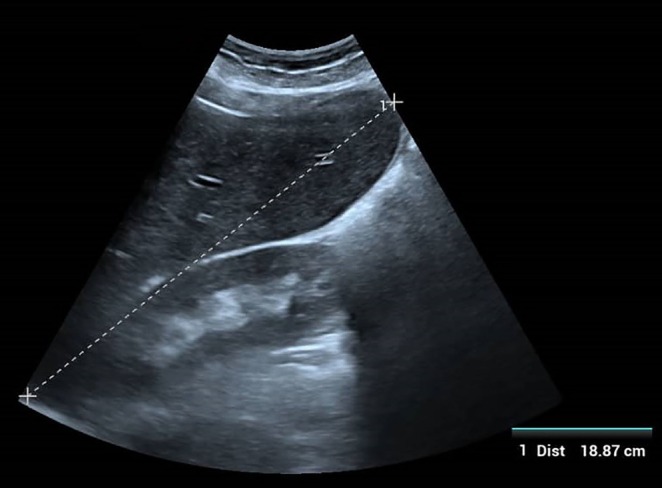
Abdominal ultrasound demonstrating hepatomegaly (liver span 18.87 cm) with homogeneous echotexture and smooth surface. No focal mass identified.
